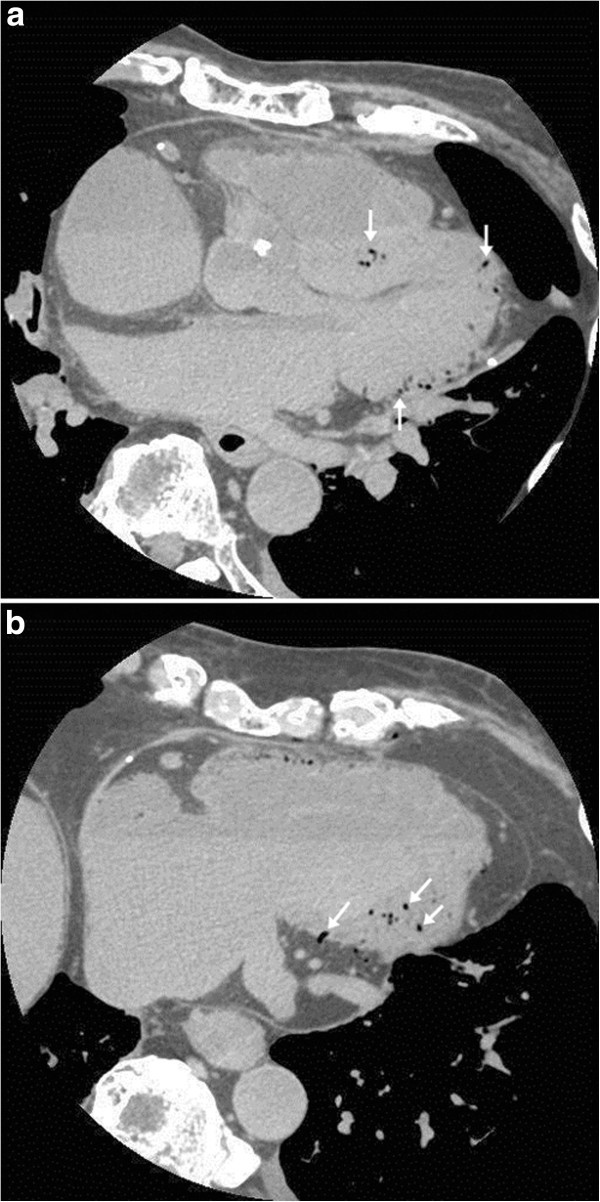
Myocardial intravascular gas of the left ventricle on postmortem CT of the heart. a. Axial image at the level of aortic root shows intravascular gas in the ventricular septum, apex, and posterior wall *(arrows)*. b. Axial image at the level of inferior wall shows intravascular gas in the inferior wall *(arrows)*.
